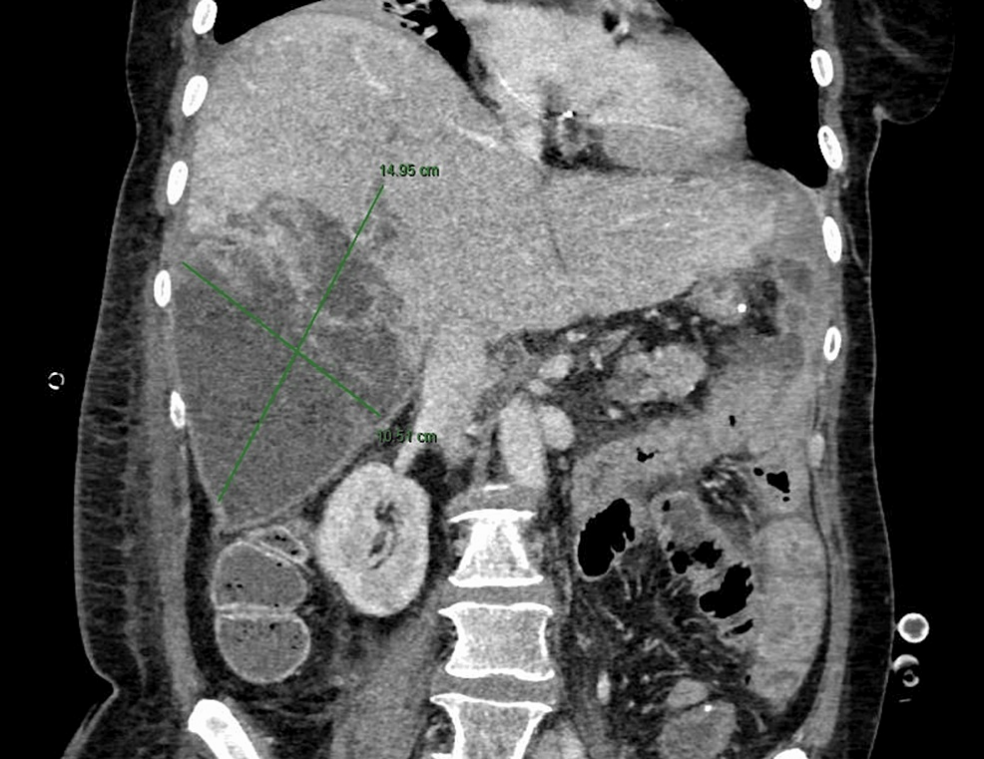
Postoperative abdominal CT scan (venous phase, coronal plane): progression of the liver metastasis in segments VI and VII, with necrotic areas inside. CT: Computed Tomography
